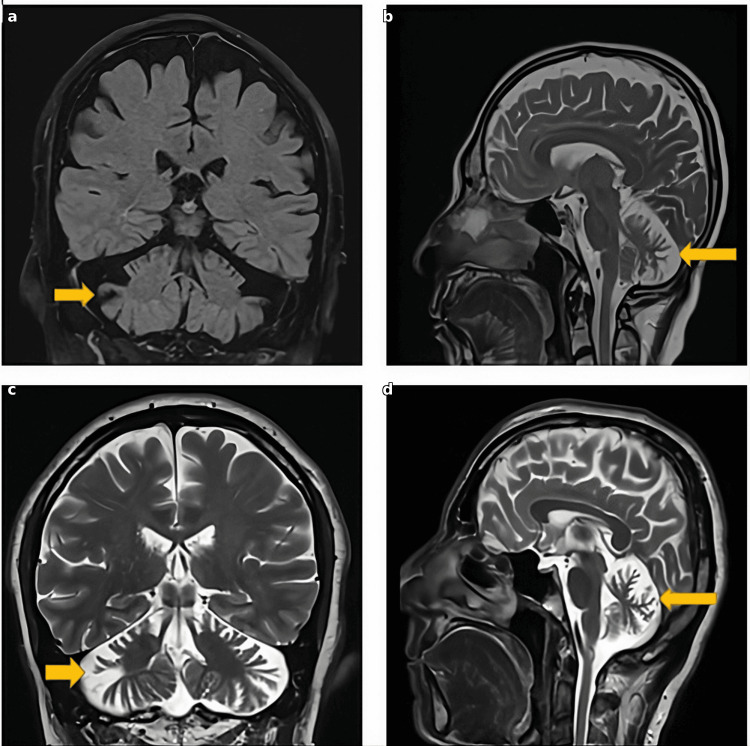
Brain MRI of Case 1 demonstrating cerebellar atrophy (a) Coronal T1-weighted image showing cerebellar vermian and hemispheric atrophy with prominence of cerebellar folia and widening of cerebellar sulci (arrow indicates cerebellar atrophy). (b) Sagittal T1-weighted image demonstrating cerebellar atrophy with enlargement of the fourth ventricle and increased cerebrospinal fluid spaces (arrow). (c) Coronal T2-weighted image showing cerebellar atrophy with prominent cerebellar sulci (arrow). (d) Sagittal T1-weighted image demonstrating cerebellar vermian atrophy with fourth ventricular enlargement (arrow).
